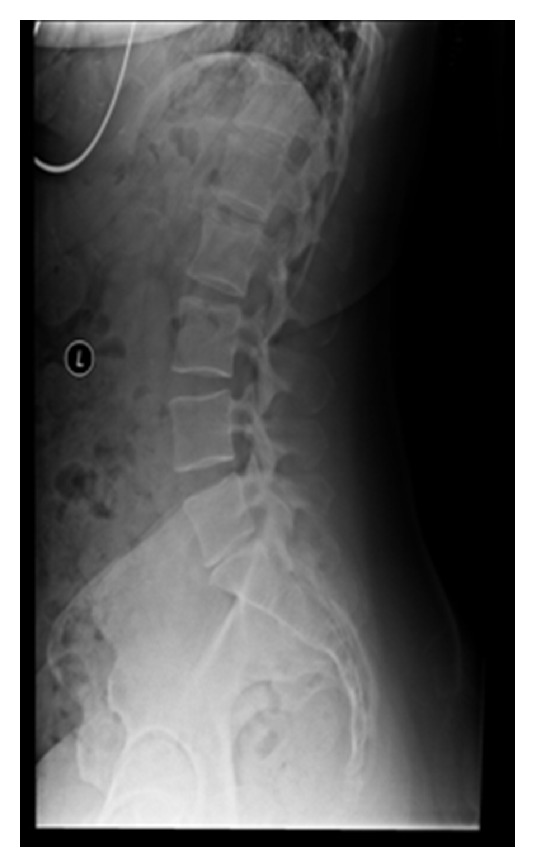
A lumbar radiograph with narrowing of the L5–S1 disc space. This narrowing is suggestive of disc degeneration.
